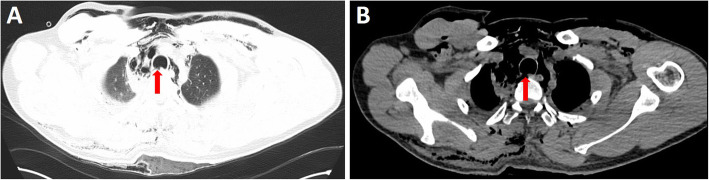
Plain chest CT revealed suspected damage to the right tracheal membrane of patient (red arrow)
